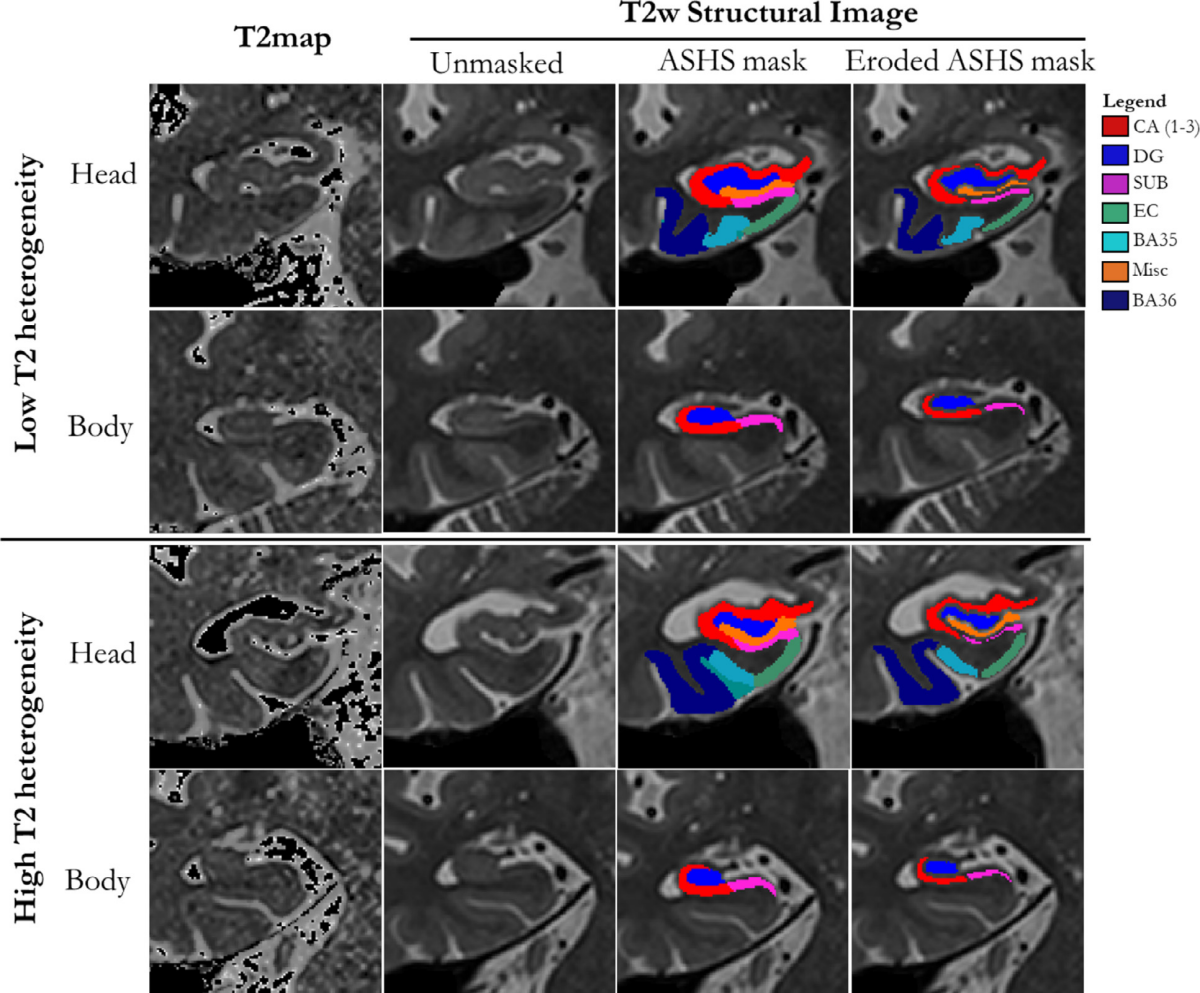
Coronal slices of MTL in two example participants with high and low T2 heterogeneity. Each row shows (left-right) T2 maps, unmasked T2-weighted structural images (summed-over-echoes), ASHS masks, and masks eroded to reduce influence of partial voluming. Top two rows show images from an individual with low T2 heterogeneity (Healthy control, age 66). Bottom two rows show images from an individual with high T2 heterogeneity (MCI, age 84). Slices from hippocampal head and body are shown for each participant. In ‘High T2 heterogeneity’ T2 maps, a higher prevalence of bright and dark spots are visible, in comparison to the ‘Low T2 heterogeneity’ T2 maps. DG = Dentate Gyrus, CA = Cornu Ammonis 1–3, SUB=Subiculum, EC = Entorhinal Cortex, BA = Brodmann Area. Misc and BA36 were excluded from all analyses. [COLOUR FIGURE].
